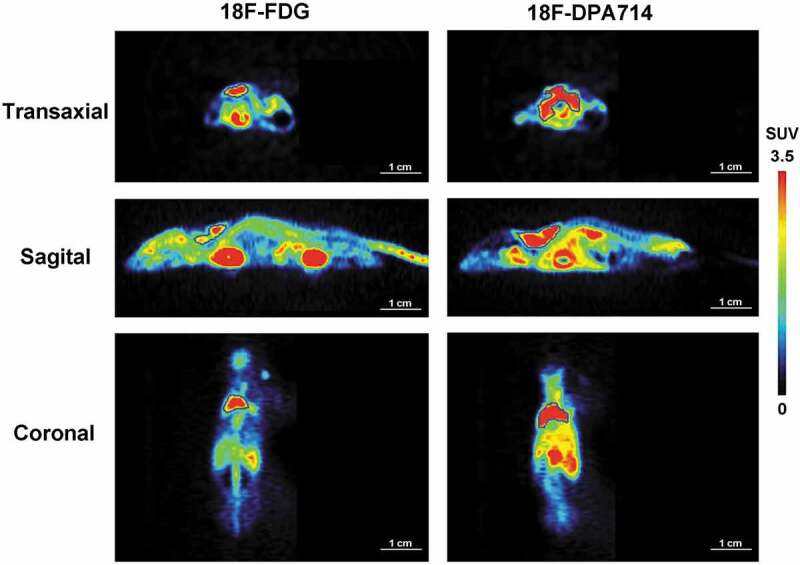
Characteristics of biodistribution of [^18^F]FDG and [^18^F]DPA714 in the CT26 tumour model
